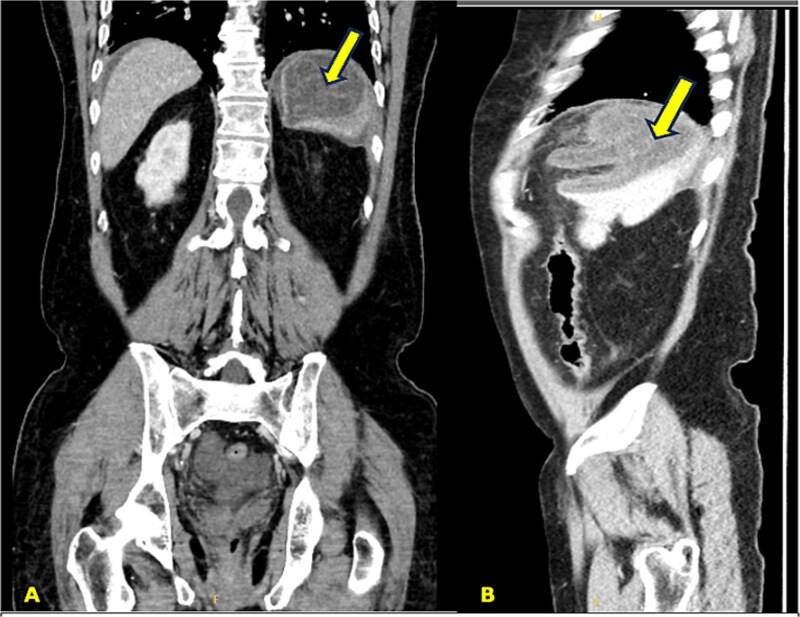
Contrast-enhanced abdominal CT images showing a large irregular cystic lesion measuring 8.0 × 15.0 × 7.3 cm in the left subdiaphragmatic region. (A) Coronal view and (B) sagittal view demonstrating an internal floating membrane. The lesion is inseparable from the splenic parenchyma, consistent with a splenic origin.
